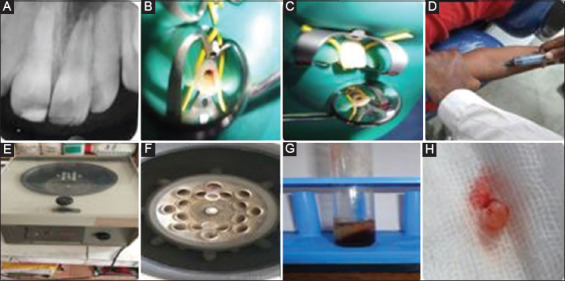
A. Pre-operative radiograph, B. Access opening, C. After calcium hydroxide removal, D. Blood drawn from anterior cubital vein, E. Centrifuge machine, F. Interior of centrifuge machine, G. Platelet-rich fibrin (PRF) in test tube, H. PRF clot.
